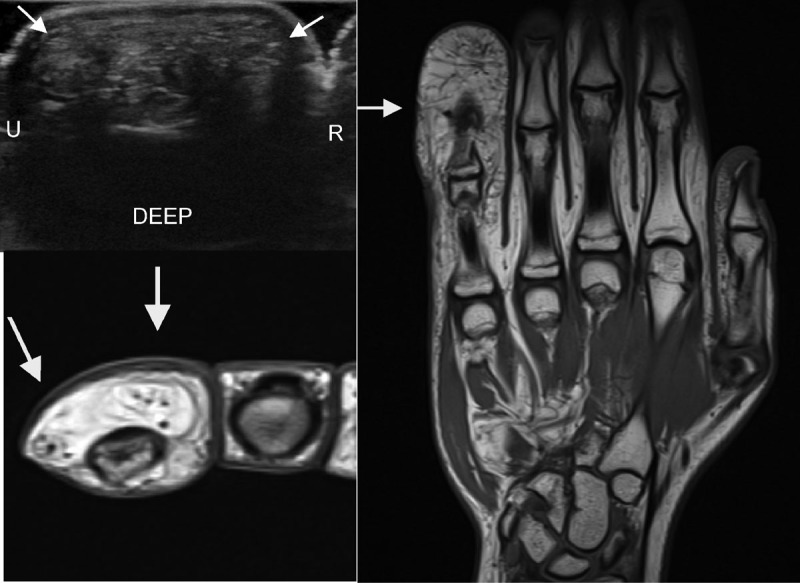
B. Patient 3. Left top and bottom image. US and MRI. Marked enlargement of the digital nerves. Note there is predominantly fatty proliferation and the coaxial cable aspect is not obvious (arrows). Right image. MRI. Marked enlargement (macrodactyly) of the little finger is seen.
